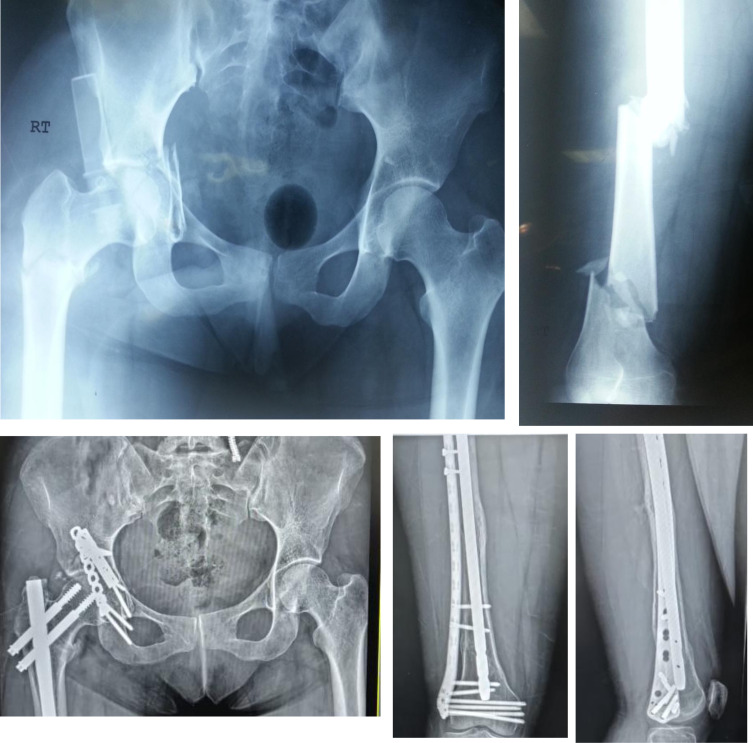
Top row: Preoperative X-rays for an ipsilateral acetabular and segmental femoral fracture, treated with fixation of acetabulum through posterior approach, and femoral nailing followed by plate augmentation for nonunion of midshaft fracture. Bottom row: 6 year follow-up X-rays.
